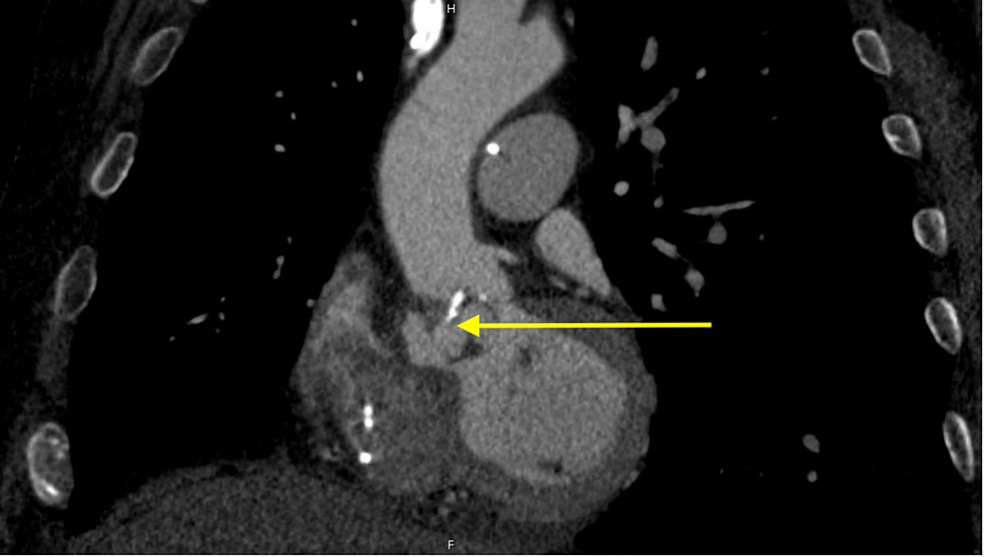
Gated coronary computed tomography showing aortic root abscess (yellow arrow).
